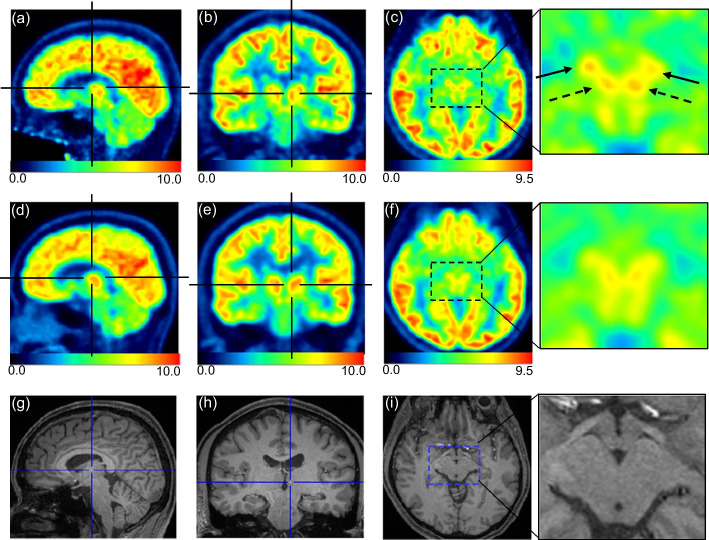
FDG-PET images using Vrain are shown at the top (a–c) and those using the whole-body PET are shown in the middle (d–f). Images g–i show the T1WI MRIs. The centre of each crossbar is placed on the anterior nucleus (a, d, g) or the dorsomedial nucleus (b, e, h) in the thalamus. These are clearly visualised using Vrain (a, b) but not using the whole-body PET (d, e). The substantia nigra (arrows) and red nucleus (dashed arrows) are visualised using Vrain (c) with an expanded scale for the squared area on the right, but each region is not visualised separately using the whole-body PET (f). The SUV is indicated by the colour scale
